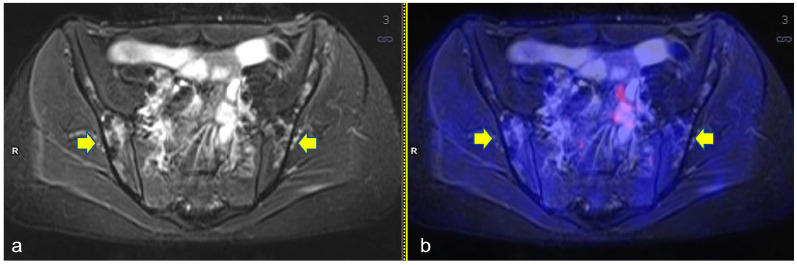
Axial turbo inversion recovery magnitude (TIRM) image (a) and fused axial TIRM and [^18^F]FDG-positron emission tomography (PET) (b) of the PET/MR scan of a 17 year-old girl affected by Hodgkin’s lymphoma, performed for re-evaluation after chemotherapy (ERA-PET), clearly demonstrating the presence of ischemic lesions in the iliac bones (yellow arrows), with no [^18^F]FDG uptake.
